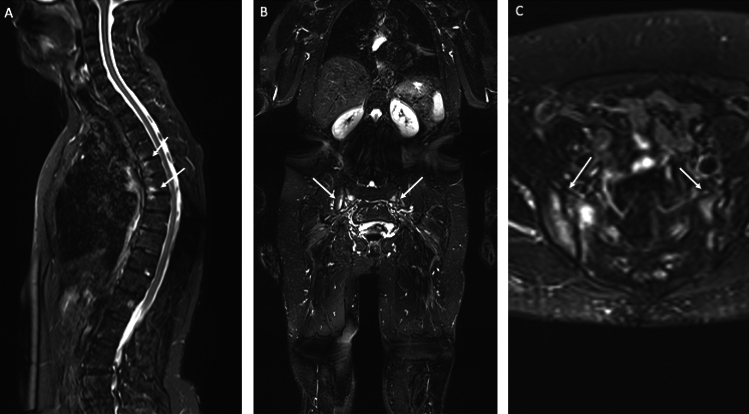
WB-MRI in a 59-year-old woman with PsA. Sagittal Whole-Spine (a) show anterior and posterior spondylitis in the thoracic spine. Coronal (b) and axial STIR (c) planes show bilateral intense sacroiliitis, mostly on the left side
